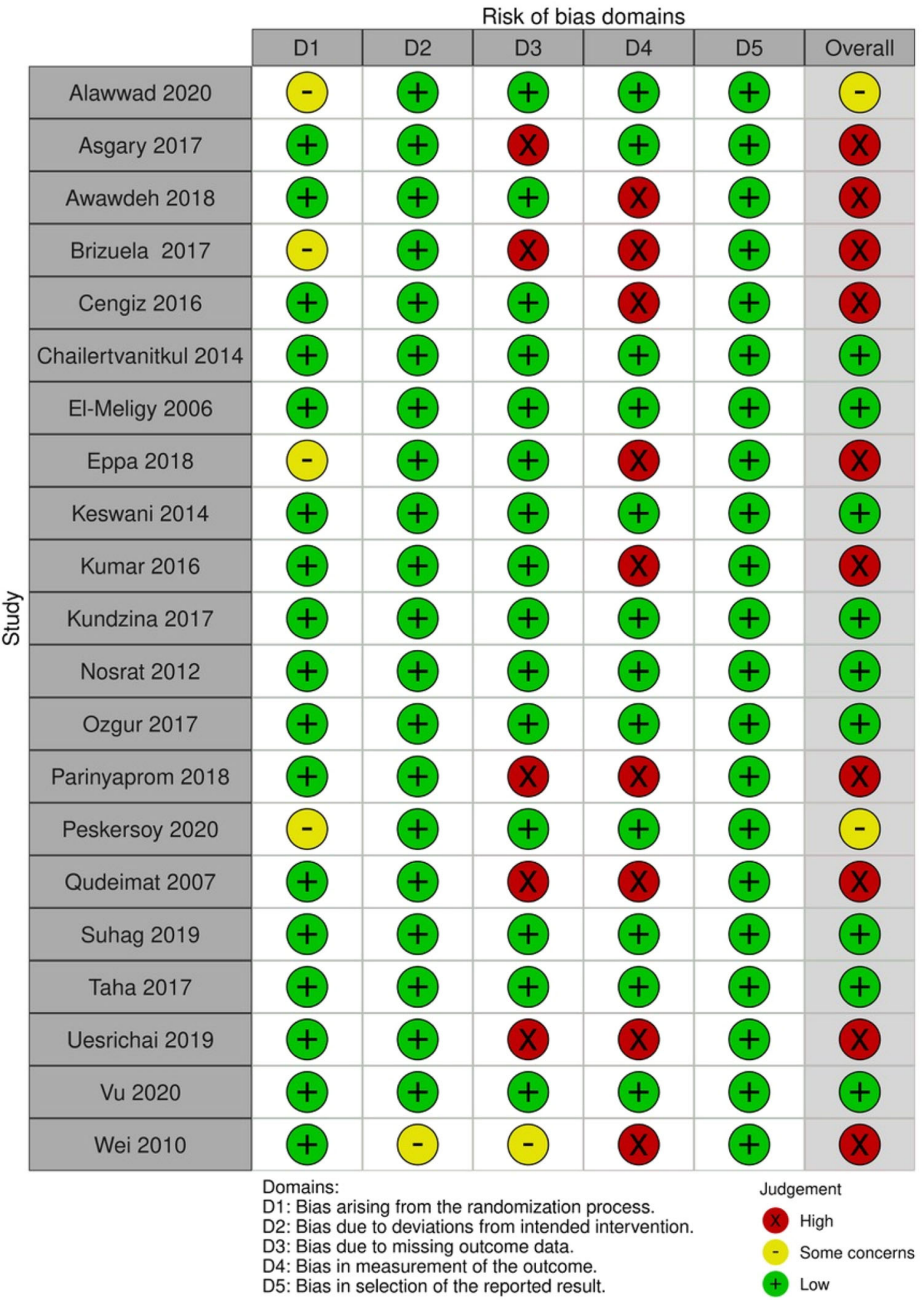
Network plot of the different capping materials for the primary outcome clinical and radiographical success. Each intervention is indicated by a node and each comparison by a link between two nodes. The size of the nodes and the thickness of the links are weighted by the number of trials investigating the corresponding interventions and comparisons, respectively. Colored templates were used to indicate multi‐arm trials. Acem, Acemannan; AR, Abscess remedy; BD, Biodentine; CEM, Calcium enriched mixture; CH, calcium hydroxide; NHA, nano‐hydroxyapatite; PRF, platelet‐rich fibrin; TAP, triple antibiotic paste.
